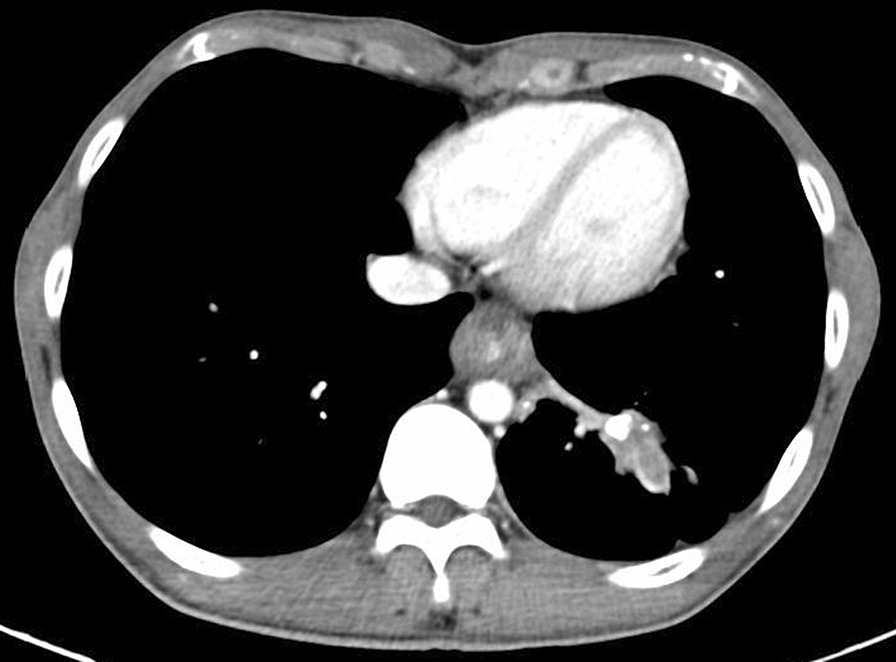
The contrast enhanced CT scan (year of 2018) demonstrated a slight increase in size of the dumbbell-shaped lesion (cross-section)
